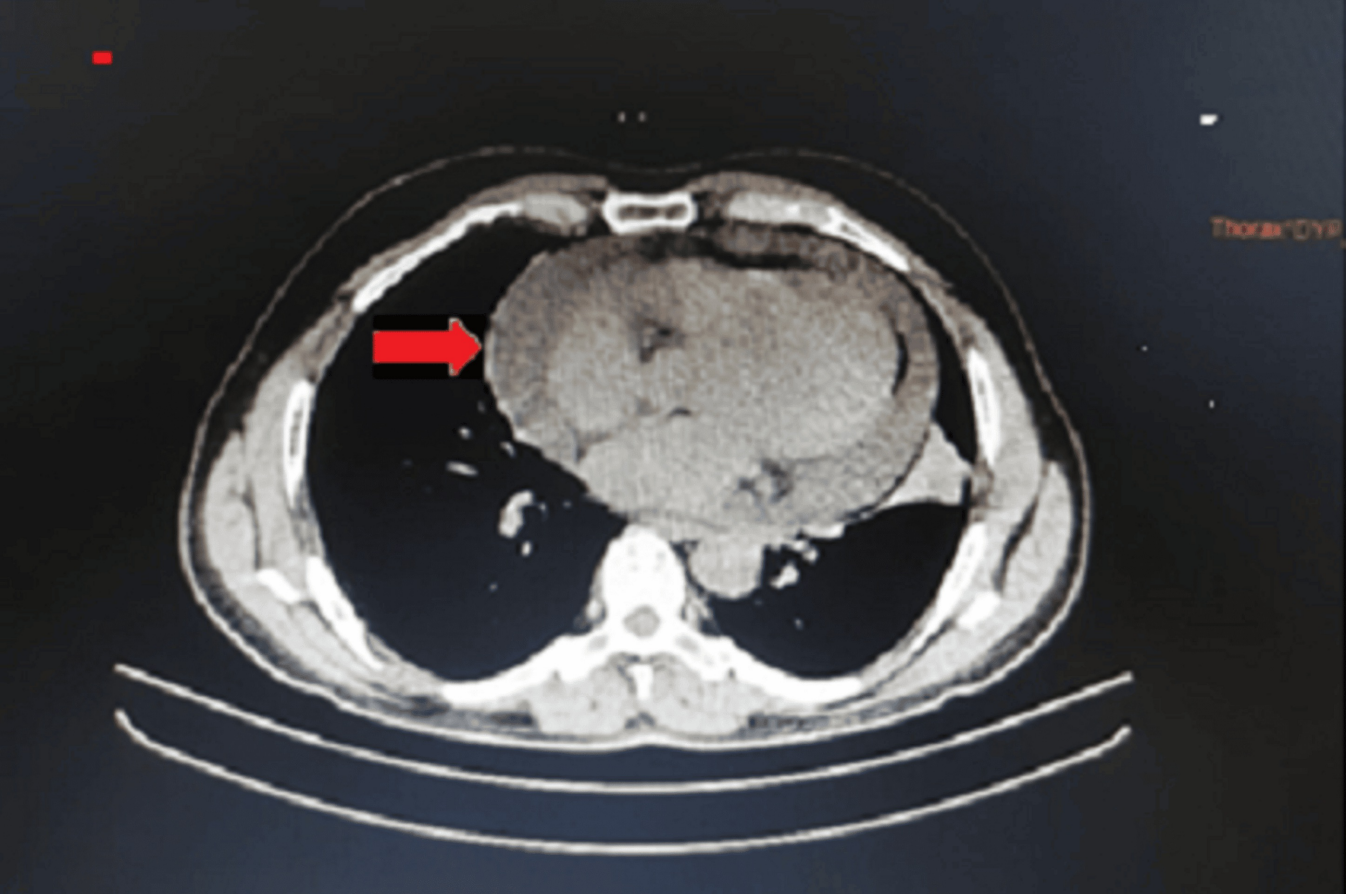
High-resolution computed tomography (HRCT) of the thorax revealed moderate to gross pericardial effusion with homogenously enhancing mass lesion
